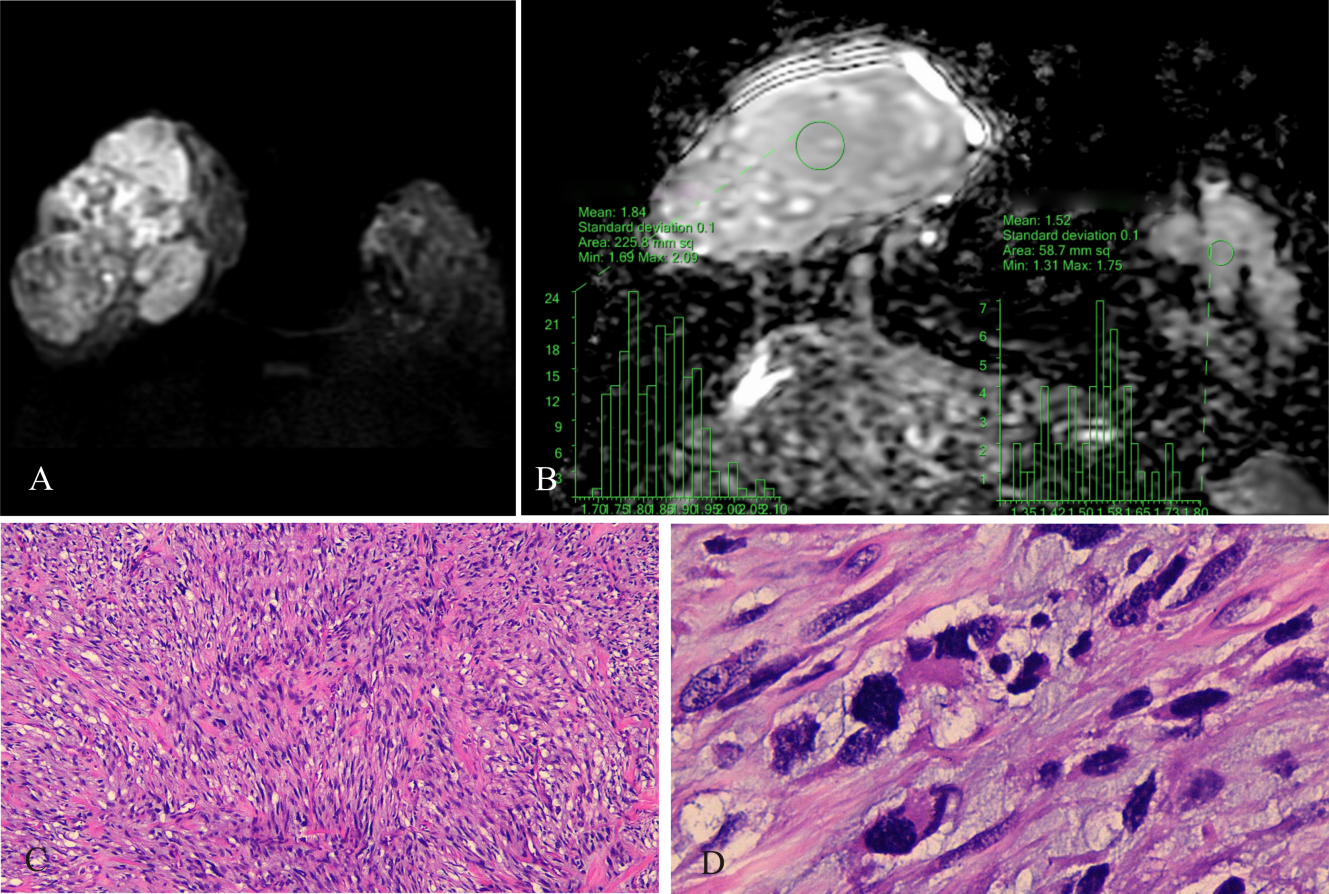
Malignant PT of the right breast, female, age 29 years. (A) DWI (b800 s/mm^2^): mixed hyperintense signal; (B) ADC map: isointense-hyperintense mixed signal, ADCmean = 1.84 × 10^−3^ mm^2^/s; ADCmean (left normal breast) = 1.52 × 10^−3^ mm^2^/s; (C) HE ×100: tumor stromal cells were closely packed; (D) HE ×400: nuclear fission, interstitial edema was insignificant.
